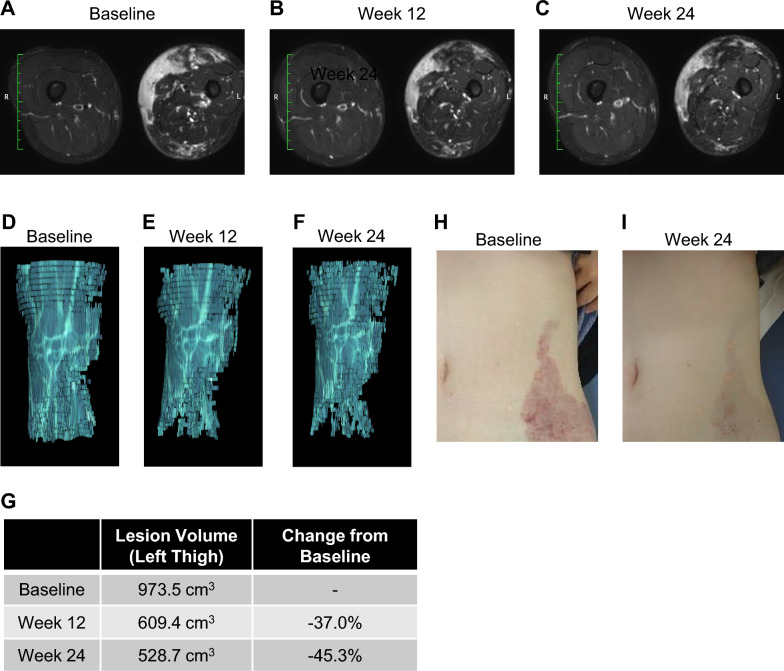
Representative radiological and clinical changes with ART-001. MR image (A–C), MR volumetry (D–F), and volume data (G) of the target lesion (left thigh), and clinical photograph (H, I) of an 18-year-old patient with KTS, who was treated with 100 mg ART-001. The data was taken at baseline (A, D, and H), week 12 (B, and E), and week 24 (C, F, and I)
